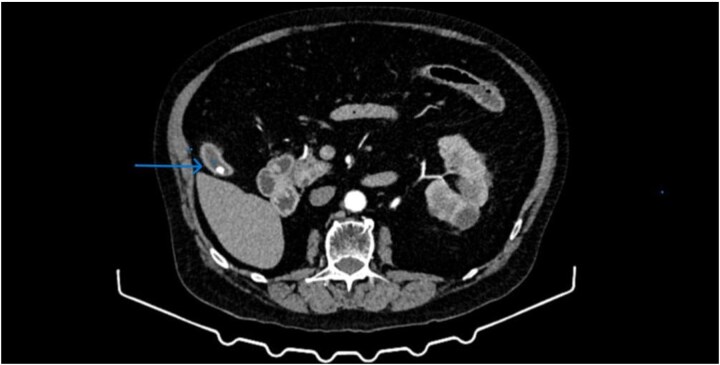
CT showed the presence of a gallbladder calculus as well as a lack of communication between the gallbladder and the hepatic parenchyma.
